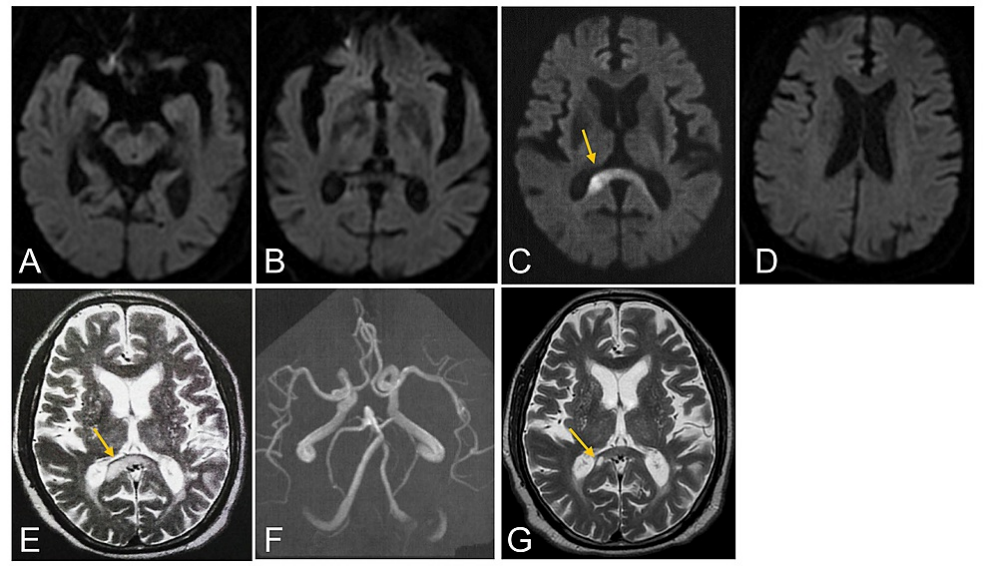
Magnetic resonance imaging Diffusion-weighted imaging on admission showed a high-intensity lesion spreading from the right-side splenium of the corpus callosum to the contralateral side (arrow in C), but did not show any other lesions in other portions of the visual pathways (A, B, D). T2-weighted imaging on admission showed the same finding (arrow in E). The magnetic resonance angiography revealed mild atherosclerosis (F). Two months later, T2-weighted imaging showed a high-intensity lesion, which became localized to the right-side splenium of the CC (arrow in G).
